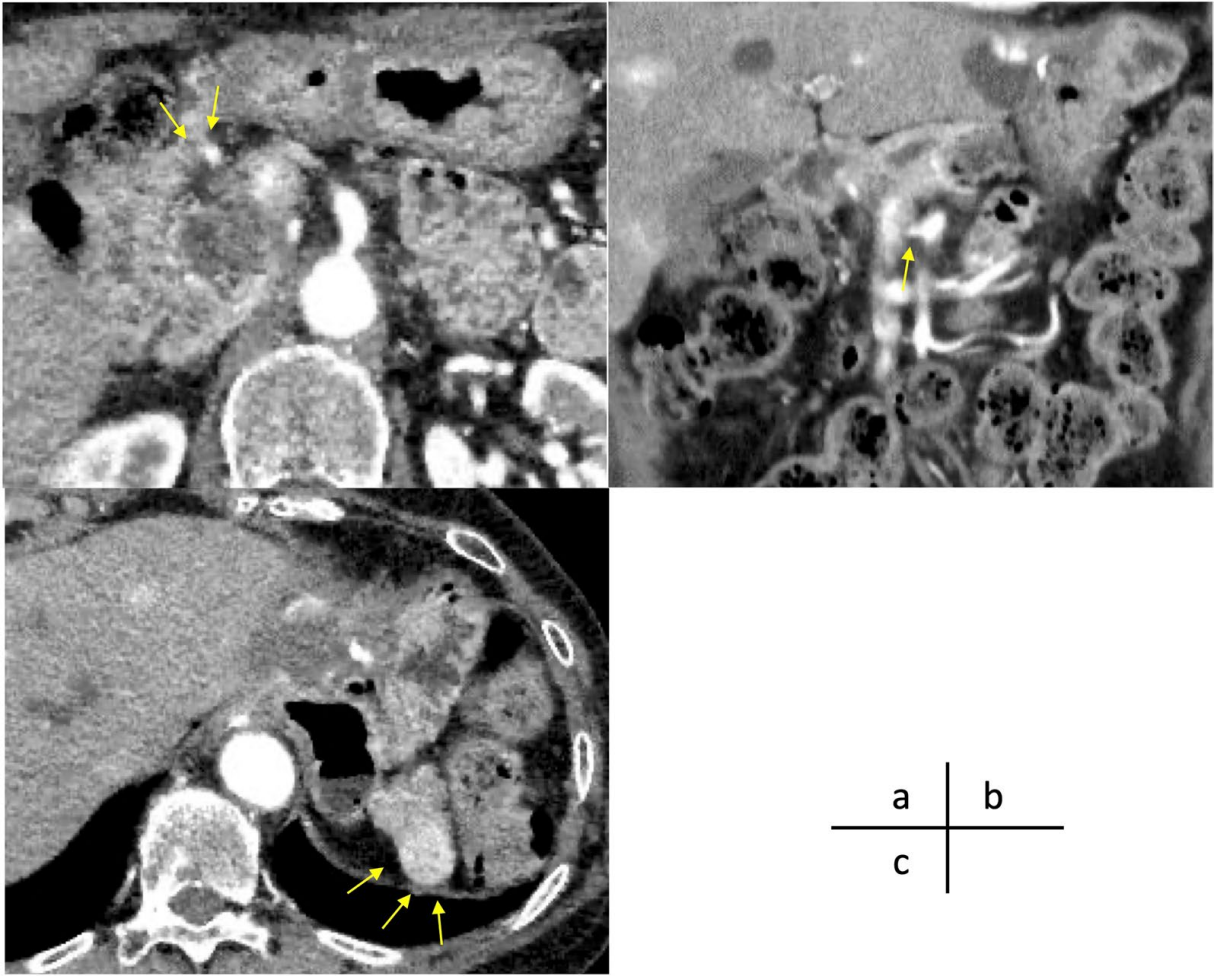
Preoperative study of computed tomography in the arterial phase. a The gastroduodenal artery (GDA) ran the surface of the pancreas (arrow). b The right hepatic artery branches from the superior mesenteric artery. c The accessory spleen at the pancreatic tail (arrow)
